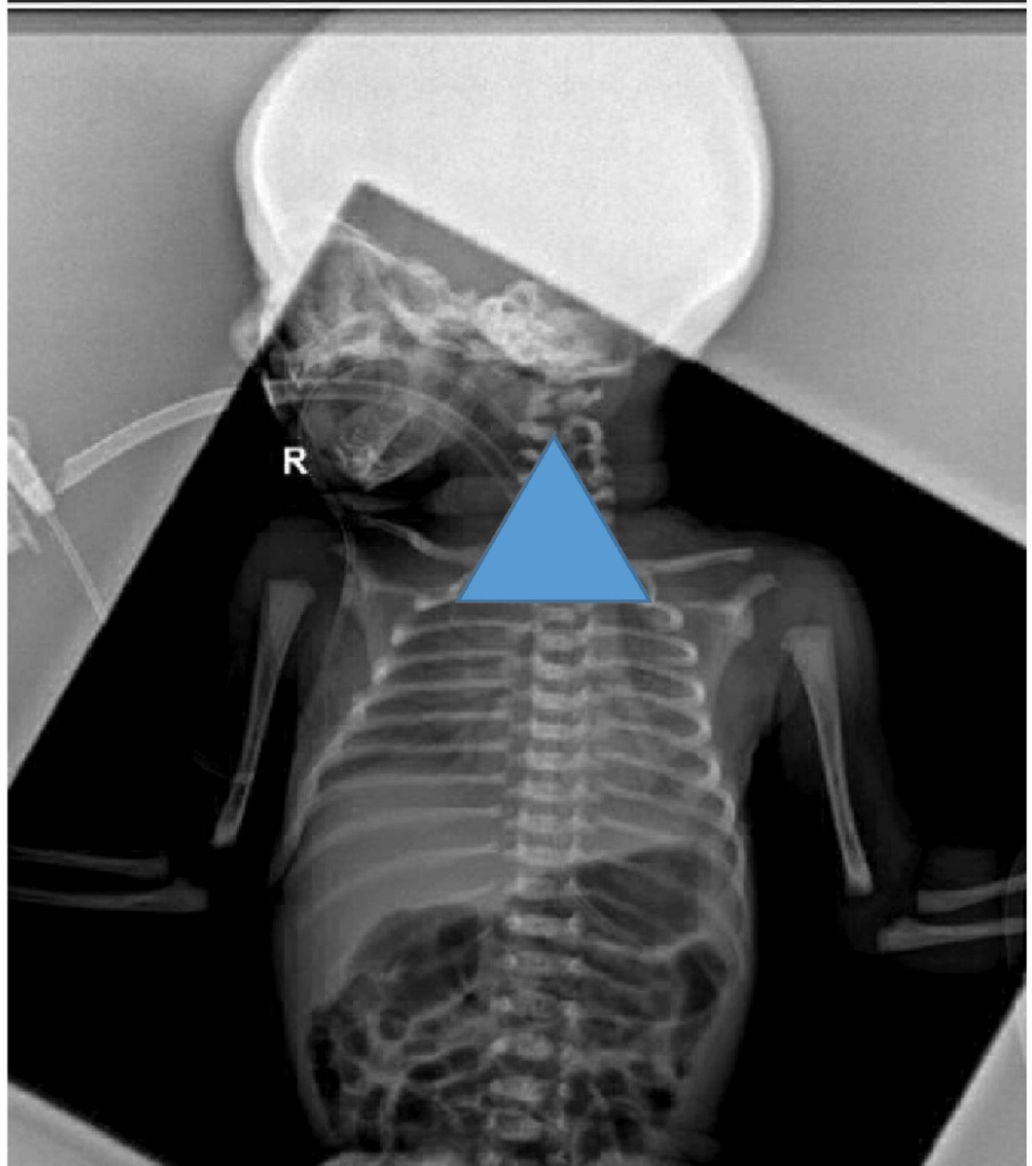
CXR image masking the ETT. CXR: chest x-ray, ETT: endotracheal tube
